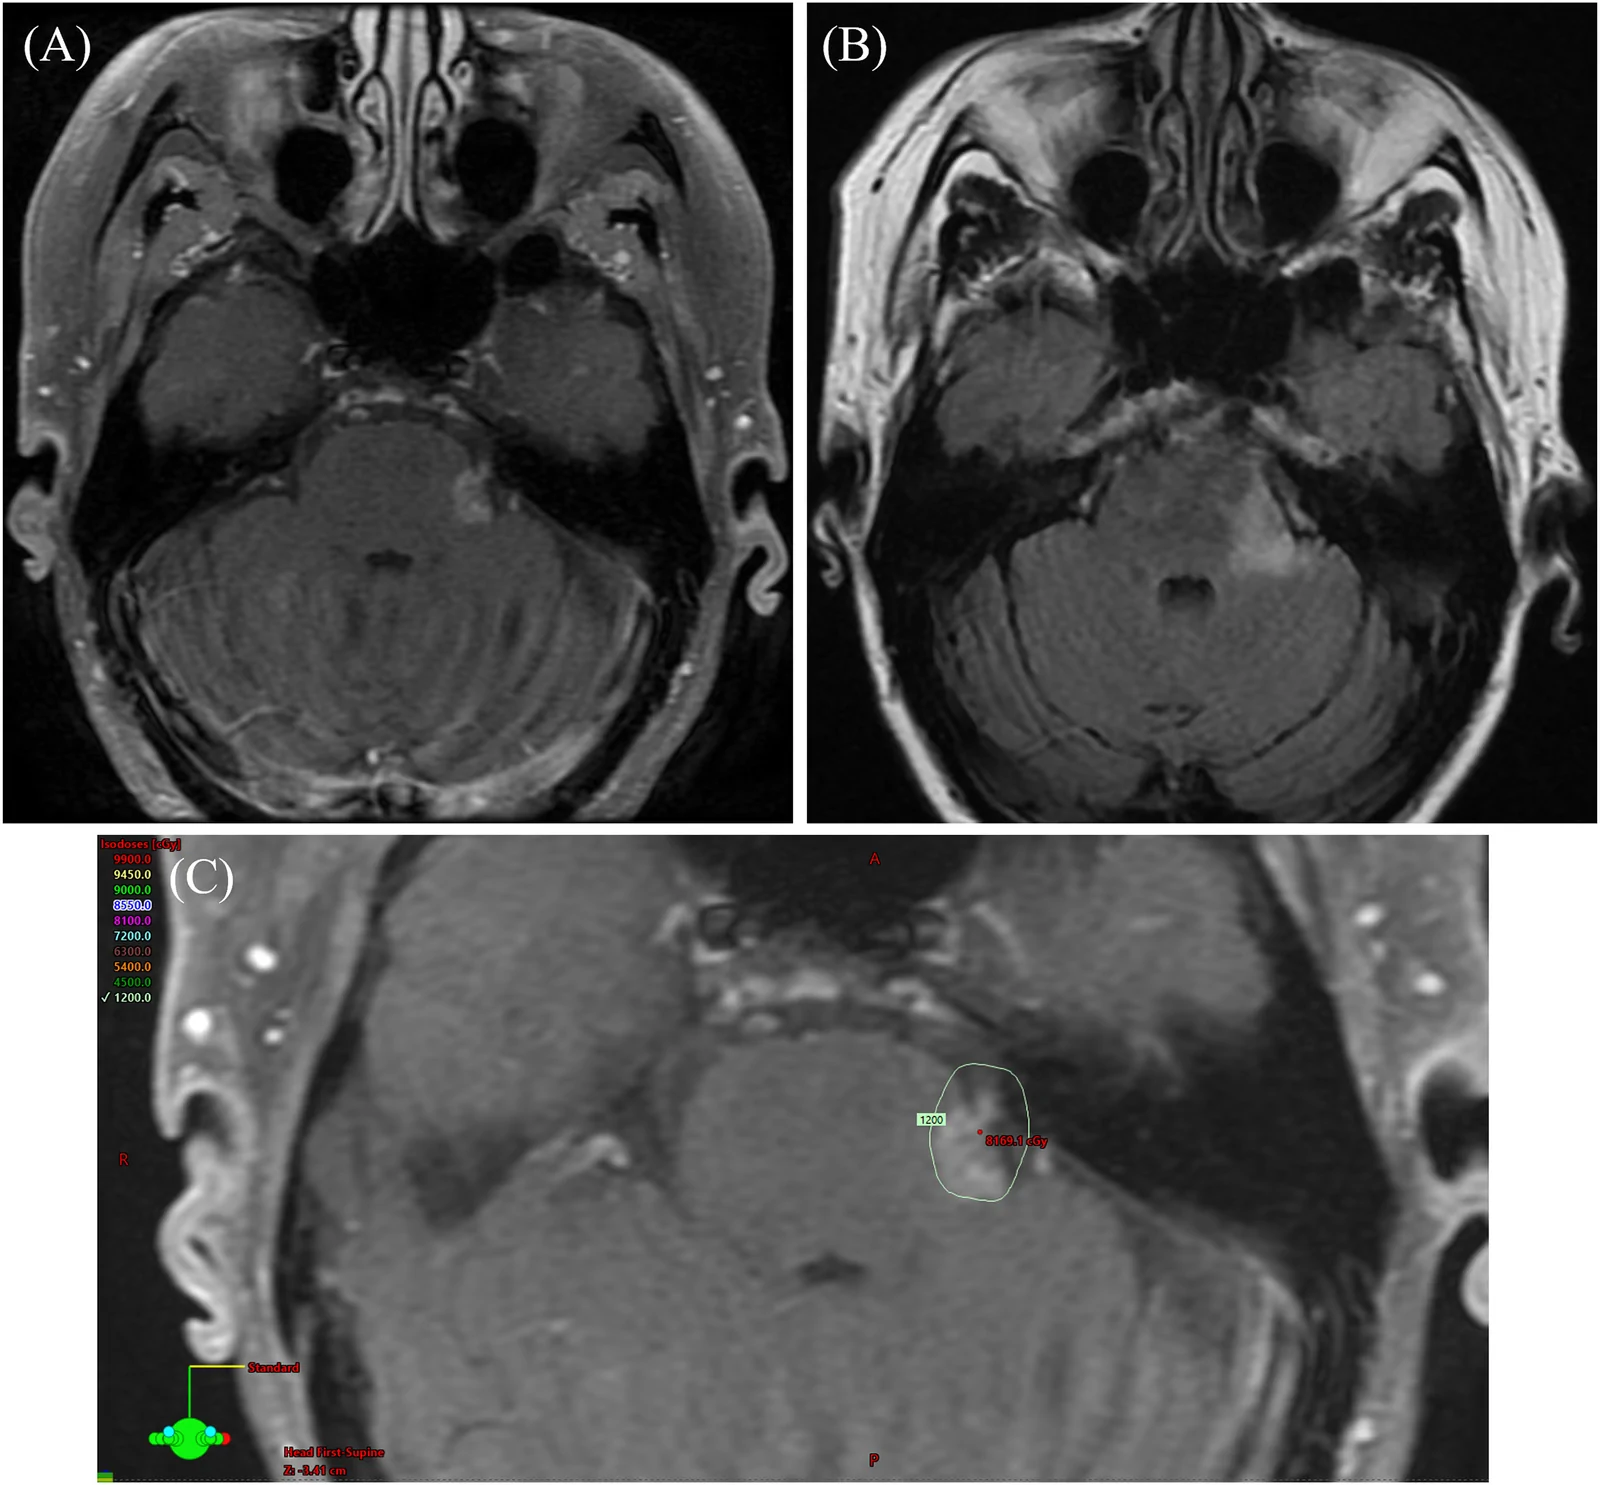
(A) Follow-up T1 magnetic resonance images with contrast yielded increased signal intensity in the lateral pons on the left side of the root entry zone. (B) Fluid-Attenuated Inversion Recovery (FLAIR) magnetic resonance imaging demonstrating greater area of enhancement and greater area of surrounding FLAIR hyperintensity at the pons root entry zone of the trigeminal nerve as well as a focal enhancement of the very proximal root of the left trigeminal nerve. (C) The T1-post and T2-FLAIR signal matched with the 1200 cGy isodose line.
